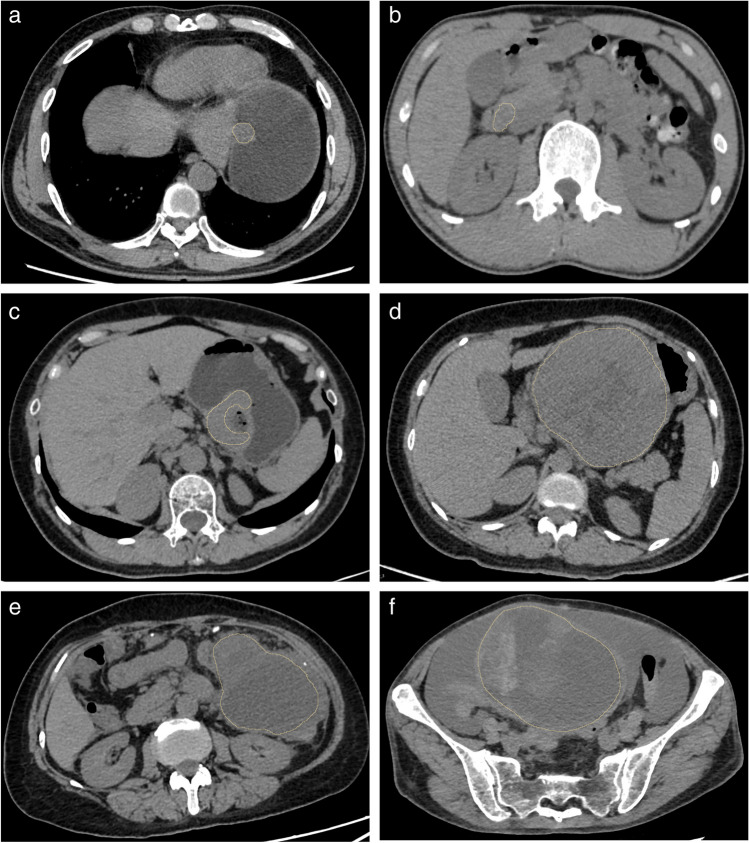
Non-contrast-enhanced CT images of patients with GISTs ordered by NGTDM Busyness. a Male, 51 years, Ki-67 PI 5%, NGTDM Busyness 9.67; b male, 27 years, Ki-67 PI 5%, NGTDM Busyness 101.66; c female, 59 years, Ki-67 PI 30%, NGTDM Busyness 1010.43; d female, 60 years, Ki-67 PI 10%, NGTDM Busyness 5823.63; e female, 61 years, Ki-67 PI 20%, NGTDM Busyness 13912.72; f female, 68 years, Ki-67 PI 30%, NGTDM Busyness 29542.20. NGTDM, neighboring grey tone difference matrix; PI, proliferation index
